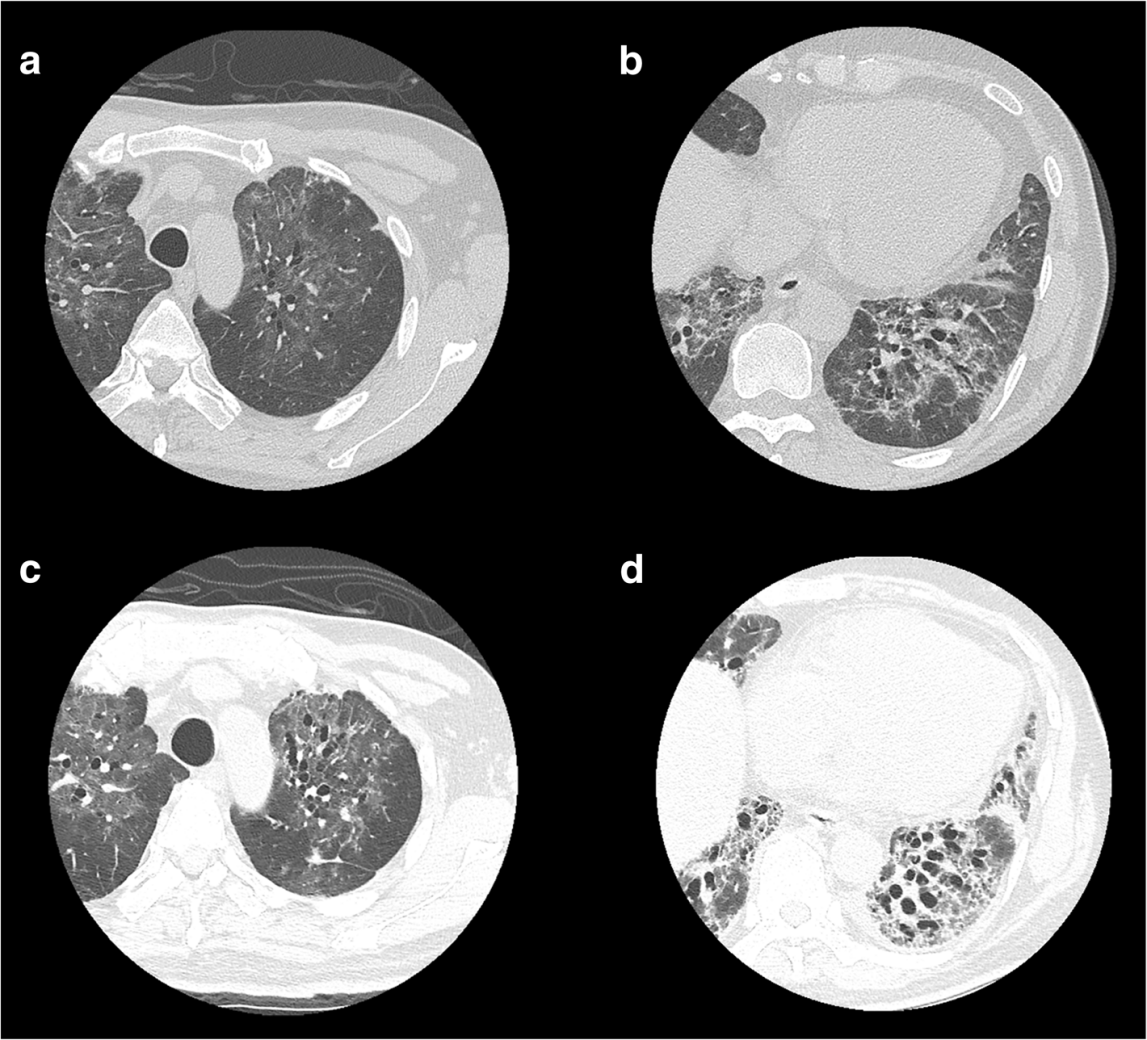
High-resolution computed tomography of the chest before (a and b, day − 56) and after (c and d, day 14) treatment of pneumocystis pneumonia
